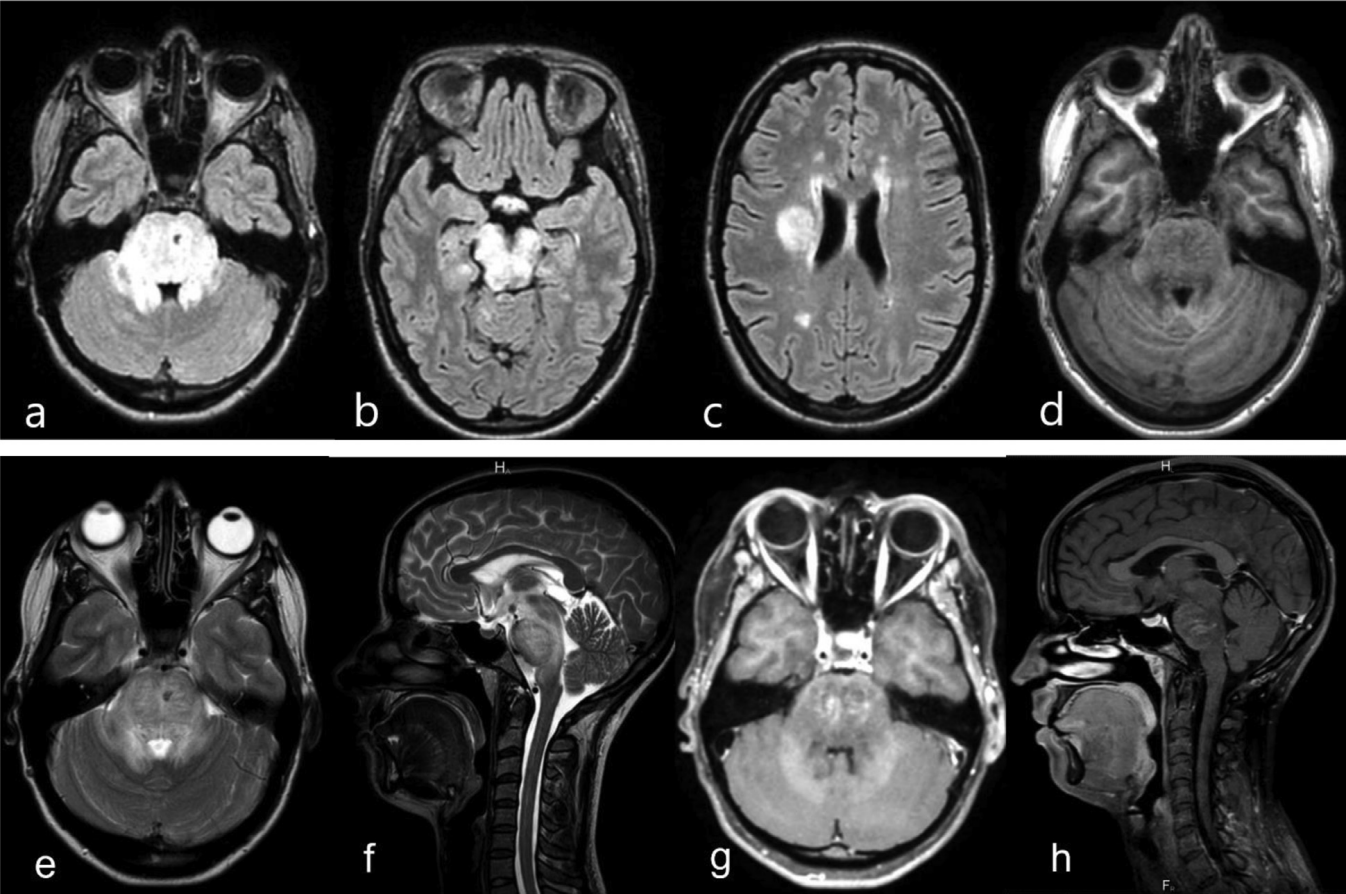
Initial representative MRI data before treatment (24 November 2021): FLAIR (a–c), T1 (d), T2 (e, f), and corresponding T1 post-gadolinium images (g, h) that resulted in a consensus diagnosis of “probable CLIPPERS.” Features of CLIPPERS pathophysiology: the T2/FLAIR high signal is much larger than enhancement, an irregular low T1 signal, associated with diffuse pontine enlargement without signs of a strong mass effect. T1 post-gadolinium data show symmetrical punctate and curvilinear enhancing lesions in the bilateral pons (arrows). No lesions or enhancing foci were noted in the cervical spine.
